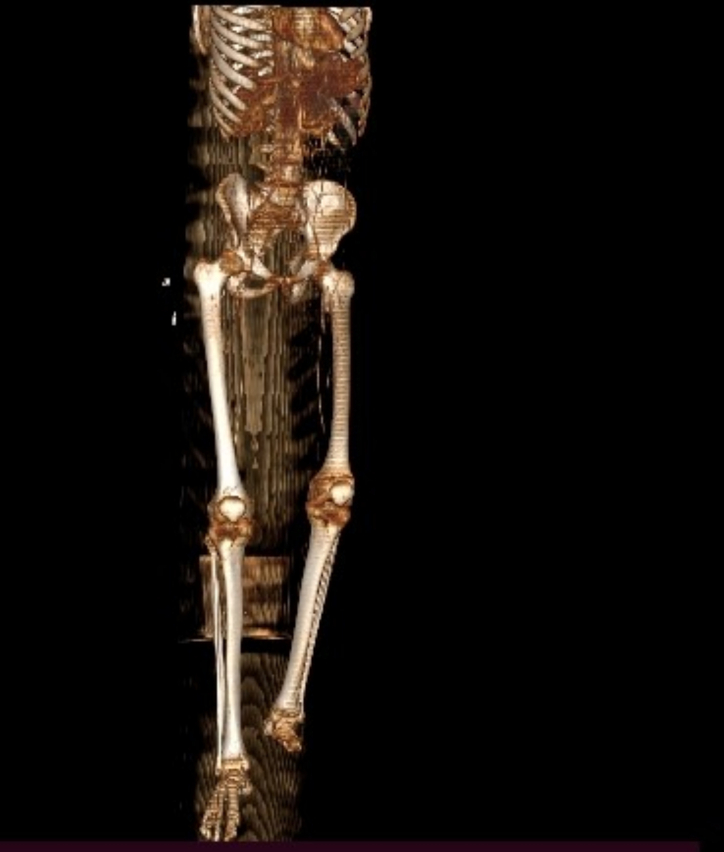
Three-dimensional radiograph image showing left mid-foot disarticulation.
